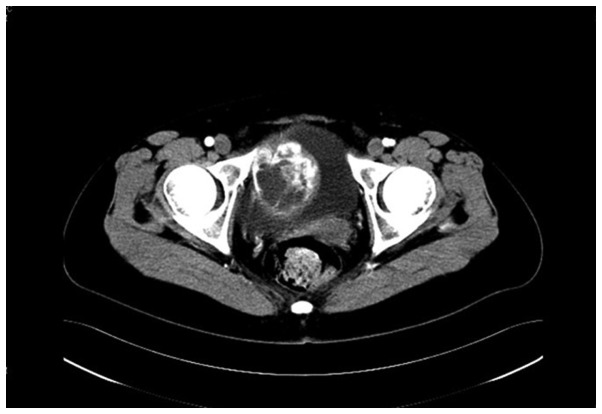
Computed tomography (CT) scan showing a well-defined heterogeneously enhancing 6.2×5.0-cm solid cystic mass in the bladder.
